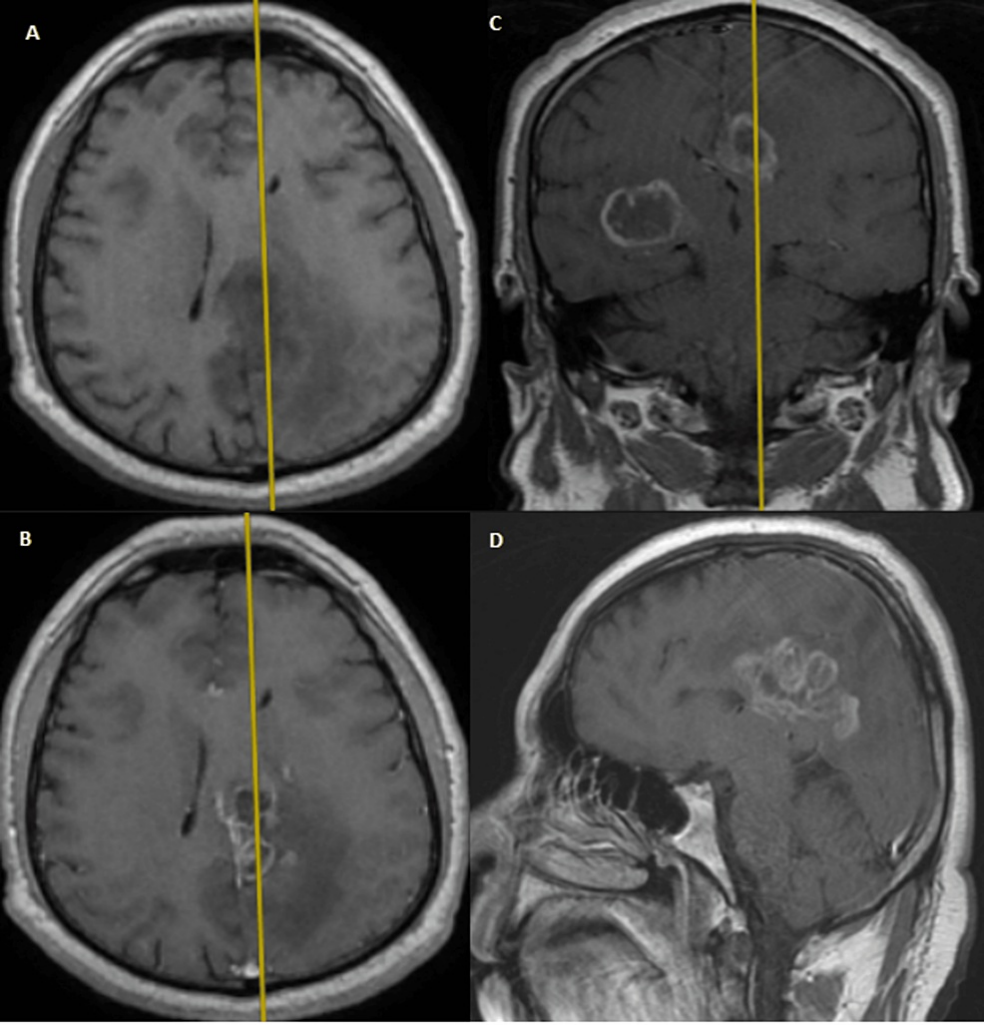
Cranial MRI showing the second lesion (parietal left-sided, all T1 sequences) (A) Axial cut without contrast; (B) axial cut with contrast; (C) coronal cut with contrast; (D) sagittal cut with contrast Image courtesy: Al Zahraa Hospital University Medical Center (ZHUMC) Radiology Department
